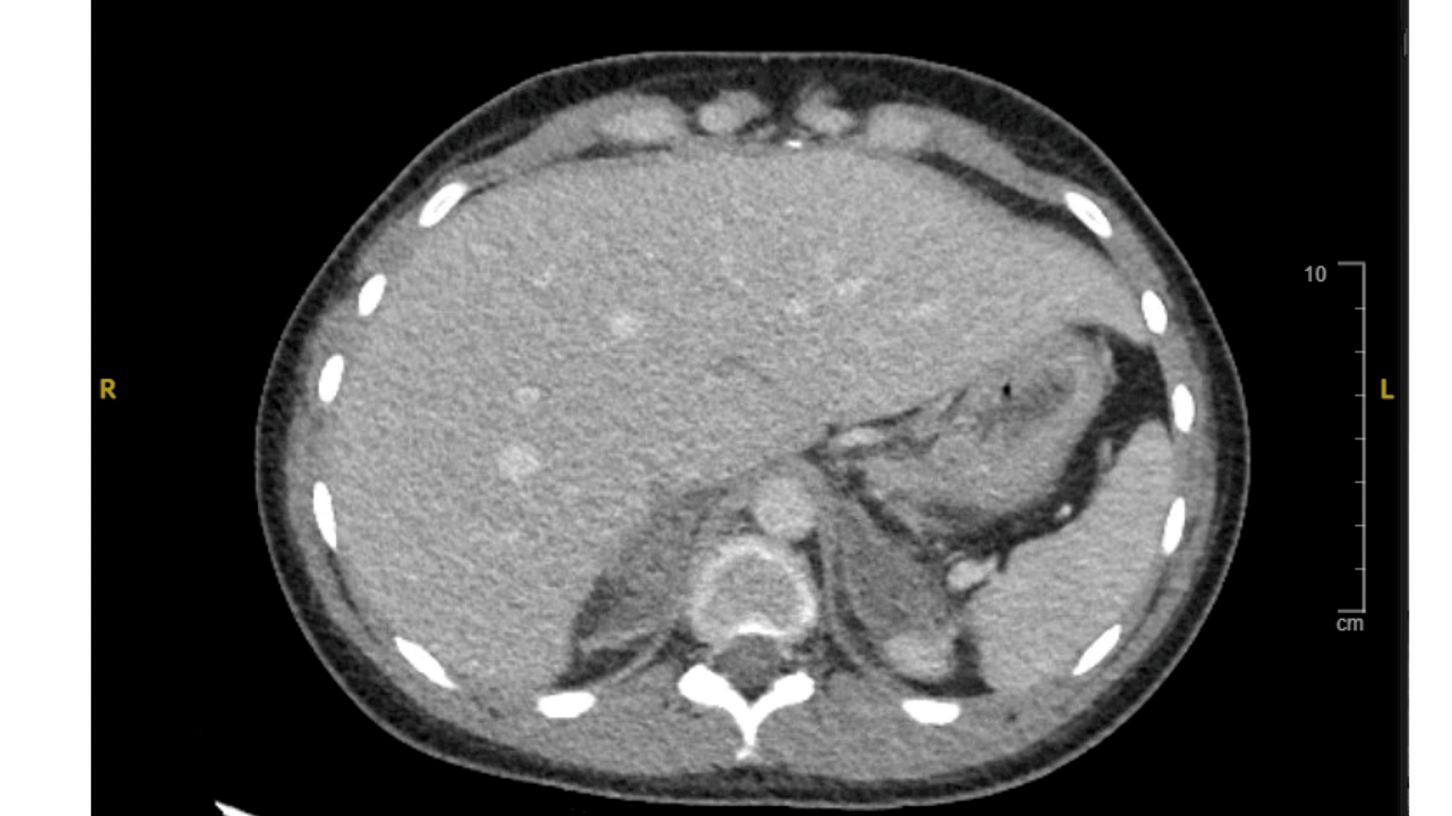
CT of the abdomen with and without contrast. There is marked adrenal gland thickening. Primary diagnostic consideration is bilateral adenomatous hyperplasia. Whether there is adrenal microhemorrhage is difficult to exclude based on the CT.
